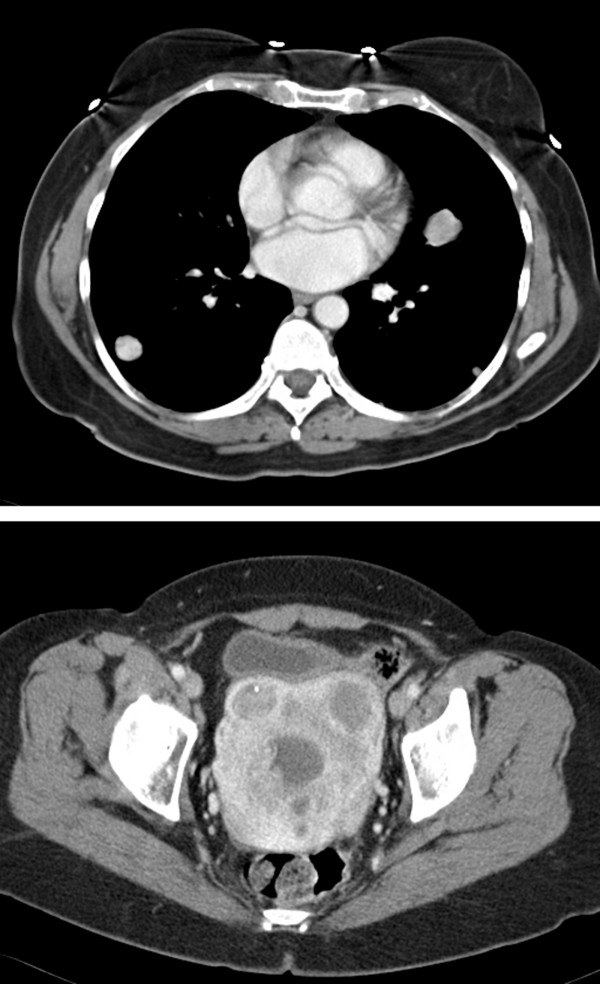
CT scan at start of chemotherapy cycle #2.
